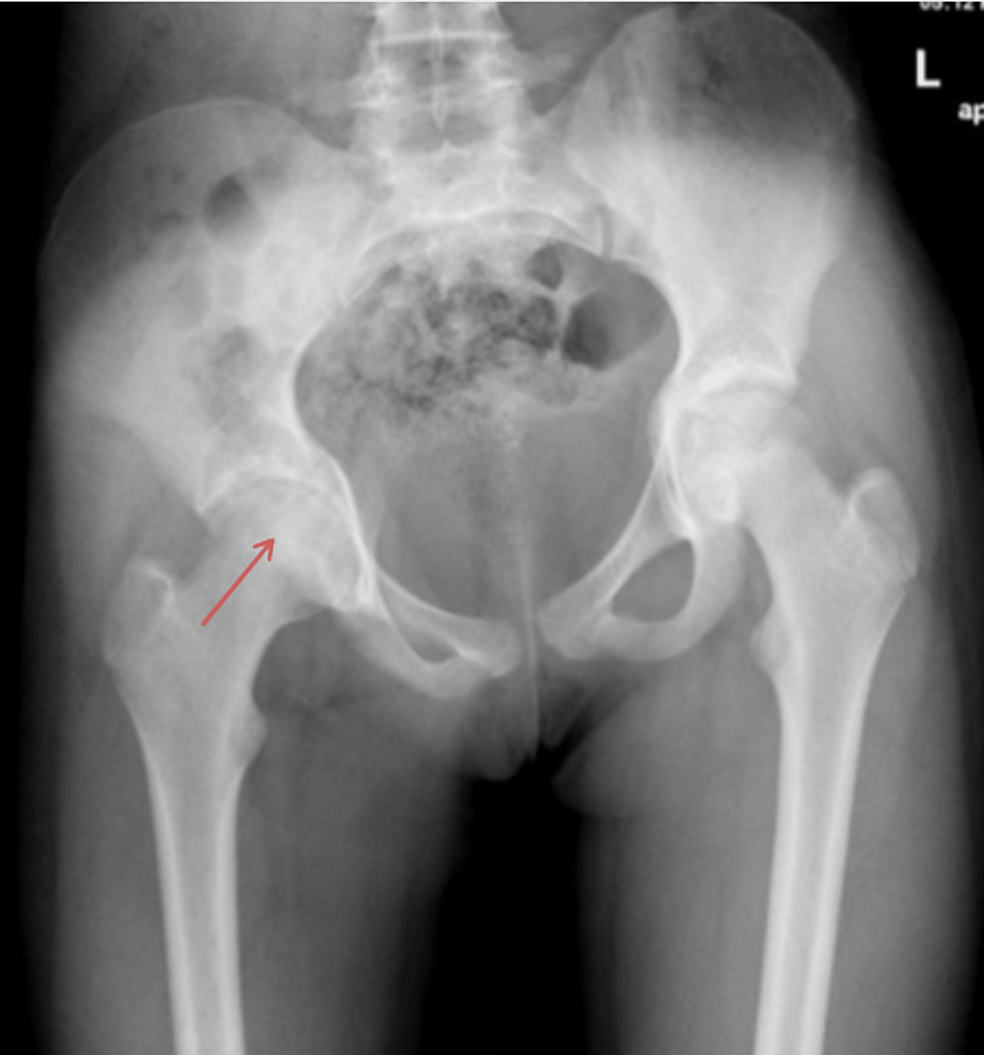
Anteroposterior pelvis radiograph showing mild medial joint space narrowing of the right hip with normal femoral head and pelvic tilt to the right side (red arrow).
